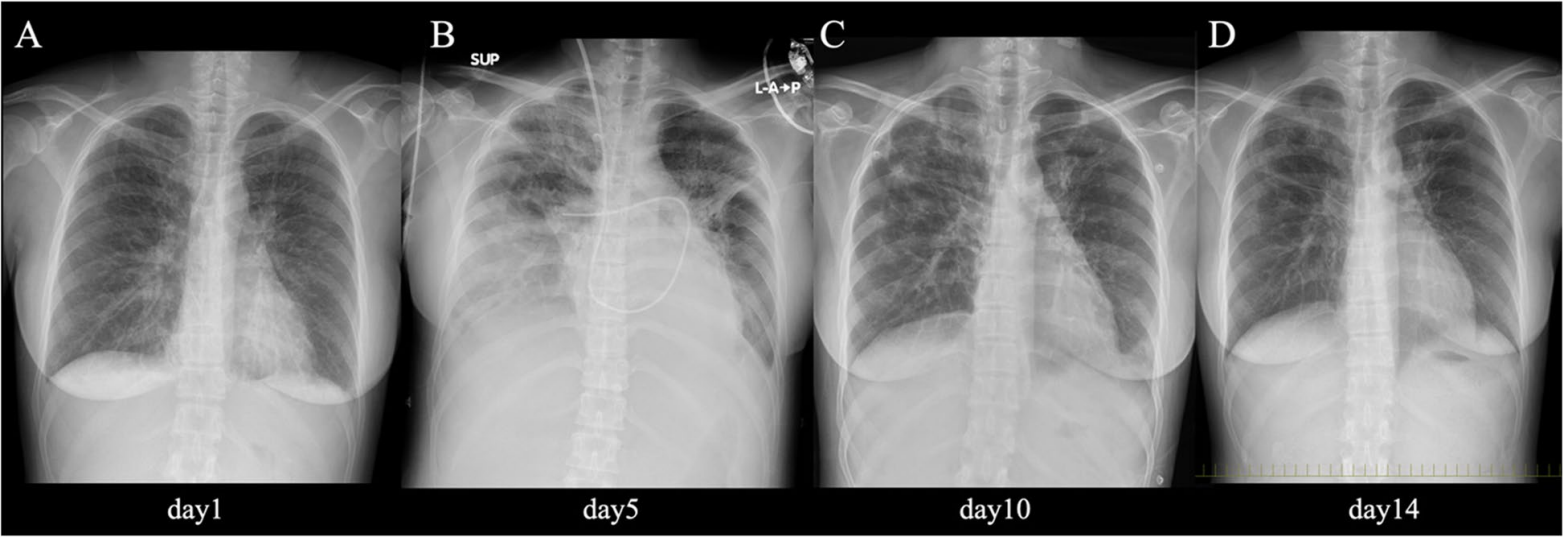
Changes in chest radiographs during hospitalization. A Day 1 chest X-ray (Upright position) revealed pulmonary vascular congestion, peribronchial cuffing, fluffy alveolar opacities, and Kerley B lines in both lungs. B Day 5 chest X-ray (Supine position) showed consolidation in both lung fields was markedly widened, and pleural effusion tended to increase, indicating exacerbation of pulmonary edema. The patient's respiratory failure worsened, and a Swan-Ganz catheter was inserted. C Day 10 chest X-ray (Upright position) showed the degree of cardiac enlargement was reduced, the enhancement of pulmonary vascular shadows was lessened, and pleural effusions decreased. D Day 14 chest X-ray (Upright position) showed that consolidation in both lung fields further improved, and the bilateral pleural effusions disappeared
